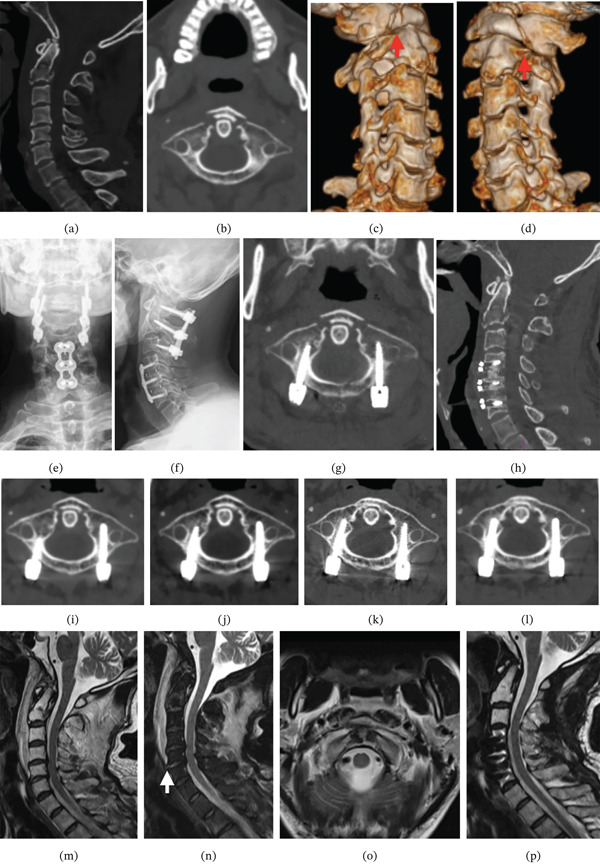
Case example (intact transverse ligament): (a–b) CT sagittal reconstructions demonstrate normal atlantodental interval, reduced basion‐odontoid distance and concurrent posterior dislocation of atlas/occiput; axial views show anterior arch fracture at the arch‐lateral mass junction with odontoid displacing anteriorly carrying an avulsed fragment. (c–d) 3D reconstructions reveal bilateral anterior arch fractures with displaced fragments (red arrows). (e–f) Postoperative radiographs: open‐mouth view shows symmetric atlantodental joints, lateral view confirms no cervical instability. (g–h) Postreduction CT: axial confirms anatomic anterior arch reduction, sagittal demonstrates restored alignment. (i–l) Serial axial CT (6/18/24/30 M) documents progressive anterior arch union with maintained normal ADI. (m–o) Preoperative cervical MRI: posterior cord displacement, hyperintense posterior atlantoaxial soft tissues, capsular ligament injury, intact transverse ligament without disruption, retropharyngeal edema. (p) Postoperative MRI: normal cord morphology without compression.
